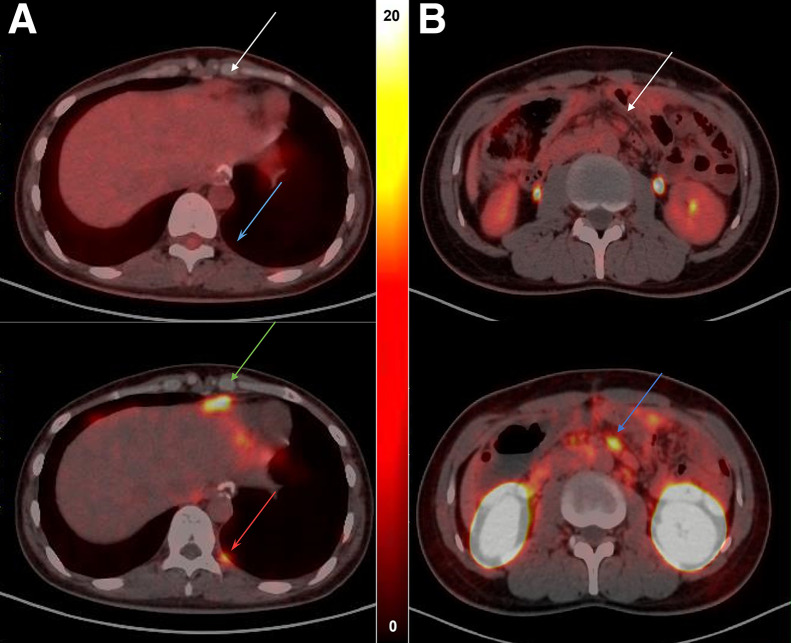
(A) Patient 9 was woman with advanced gastric cancer whose CLDN18.2 expression level was 40%, 2+. Left pleural metastatic nodule (blue arrow) and thickened peritoneum (white arrow) did not show obvious uptake on ^18^F-FDG PET/CT, whereas ^68^Ga-NC-BCH PET/CT showed high uptake (SUV_max_, 3.1 [red arrow] and 5.7 [green arrow]). (B) Patient 4 was man with colon cancer whose CLDN18.2 expression level was 40%, 3+. On ^18^F-FDG PET/CT, abdominal metastatic lymph node (white arrow) showed mild uptake, whereas same lymph nodes on ^68^Ga-NC-BCH PET/CT (blue arrow) showed high uptake (SUV_max_, 1.4 vs. 5.6).
